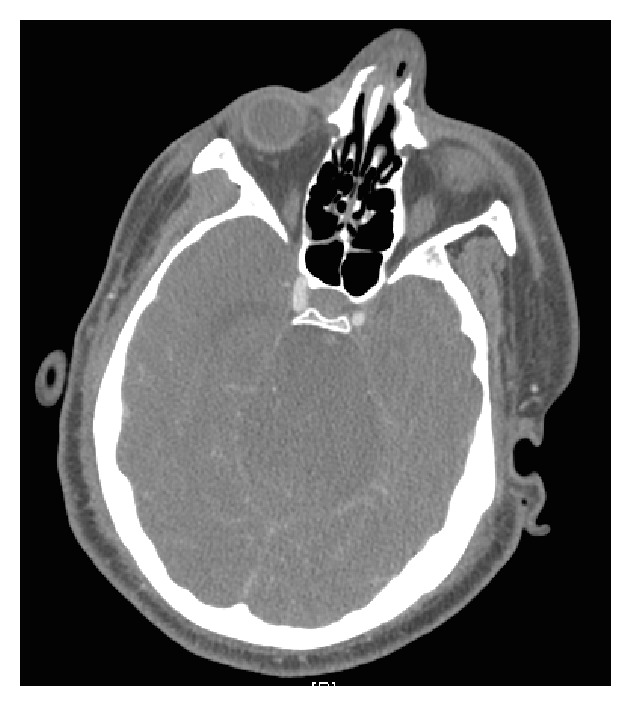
Head CT scan showing brainstem edema after infarct.
